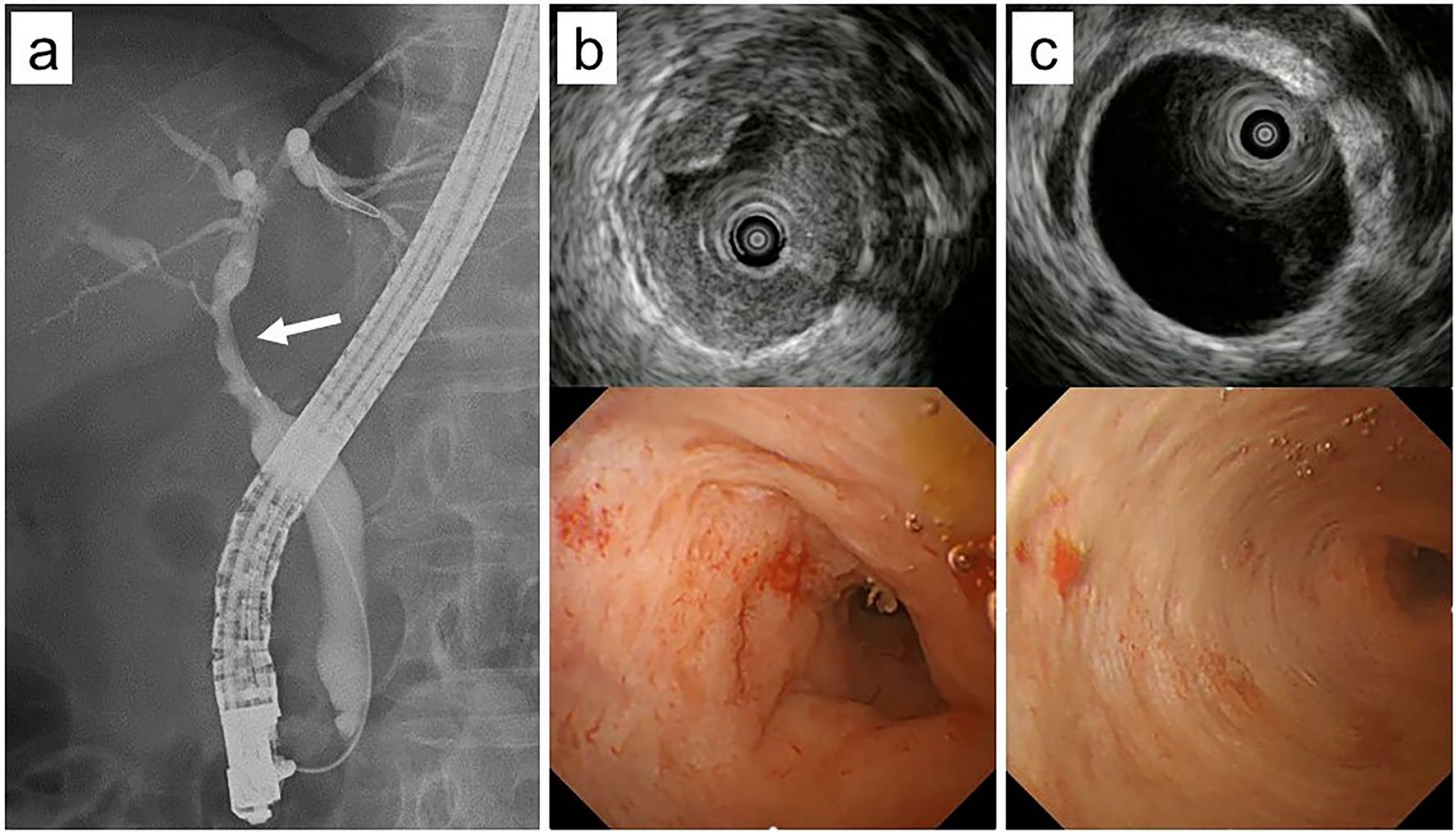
Endoscopic retrograde cholangiopancreatography. a A relatively extensive, gently rising stricture is observed in the perihilar bile duct (arrow). b In the stenotic area, intraductal ultrasonography reveals a homogeneous wall thickening of 3.5 mm and peroral cholangioscopy shows submucosal tumor-like-circumferential narrowing with mildly irregular and uneven but smooth mucosa, and no severe mucosal irregularities or unevenly dilated blood vessels suggestive of cholangiocarcinoma were observed. c In the non-stenotic area, intraductal ultrasonography reveals no wall thickening and peroral cholangioscopy shows no mucosal irregularity
